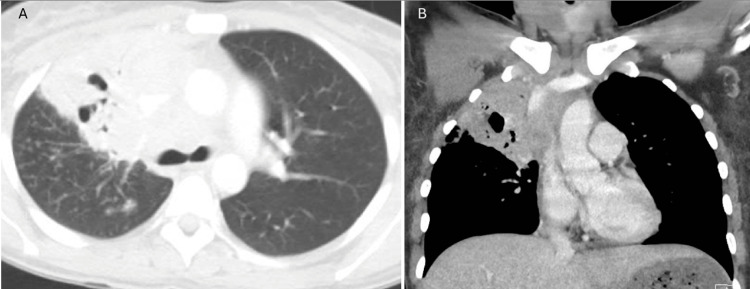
CT scan lung window axial view (Panel A) showing right upper lobe involvement with air bronchograms, and mediastinal window in coronal view (Panel B) showing soft tissue involvement within the mediastinum and right hilar region.
